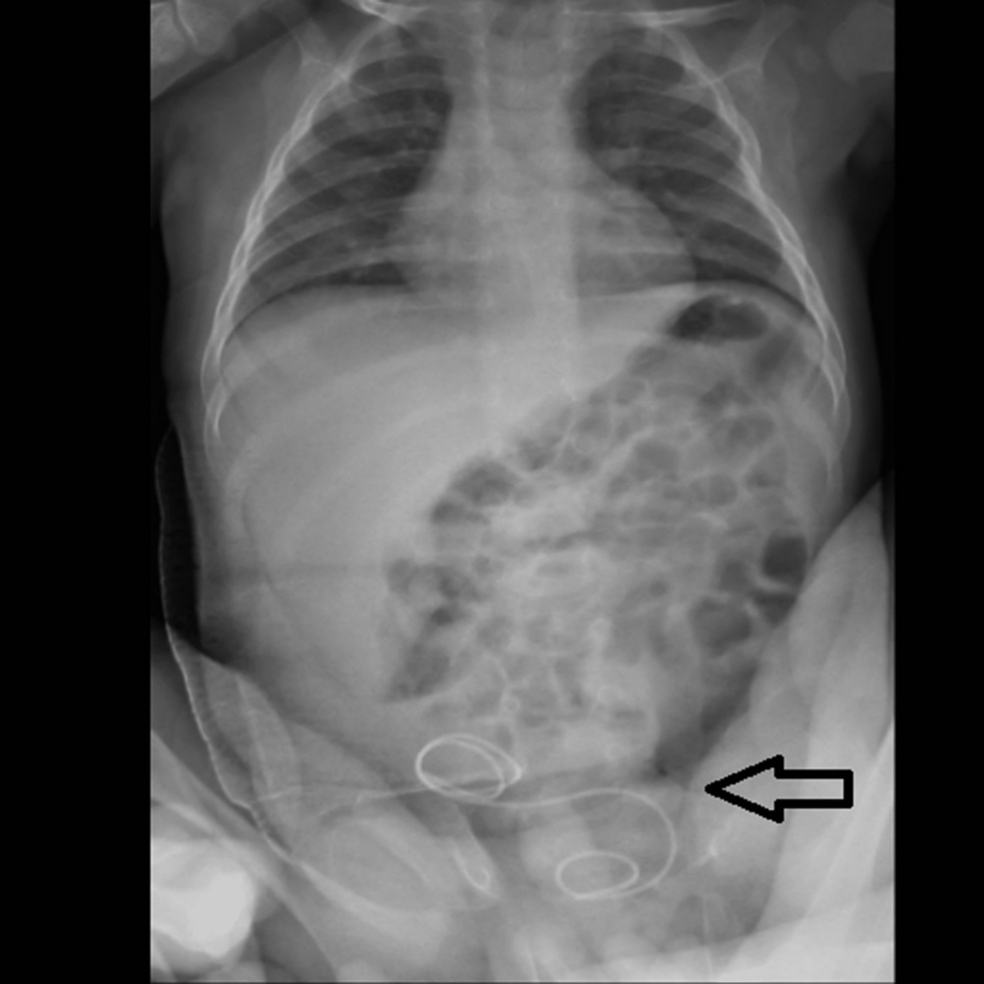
X-ray of the erect abdomen showing a 3/12 DJ stent in situ postoperatively.
